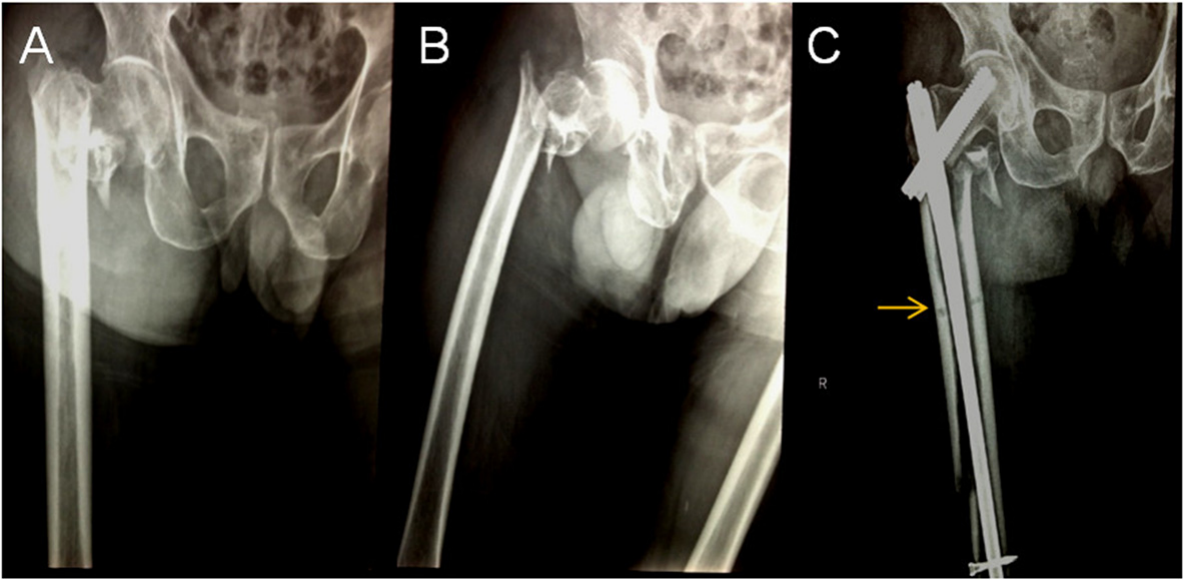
Preoperative anterior, posterior, and lateral x-ray image of the injured hip fixed with INTERTAN™ nail resulted in an iatrogenic fracture then fixed with a long and short INTERTAN™ nail to fix the iatrogenic fracture. (A) Preoperative anteroposterior X-ray image of the injured hip. (B) Preoperative lateral X-ray image of the injured hip. From the preoperative radiographs, the width of the proximal femoral canal was measured at around 11mm. (C) We did not expect there would be difficulties in the implantation of 10mm-diameter INTERTAN™ nail during the preoperative measurement of the femoral medullary cavity. After routine proximal canal reaming, we implanted the main pin manually and encountered resistance. We then hammered the main pin into the ideal position and performed the remaining steps. When the final X-ray was taken to confirm the position, an iatrogenic fracture of the distal femur was observed. The original short TRIGEN INTERTAN nail and the captured locking screw were removed (arrow). Subsequently, a longer INTERTAN™ nail was implanted to fix the iatrogenic fracture.
